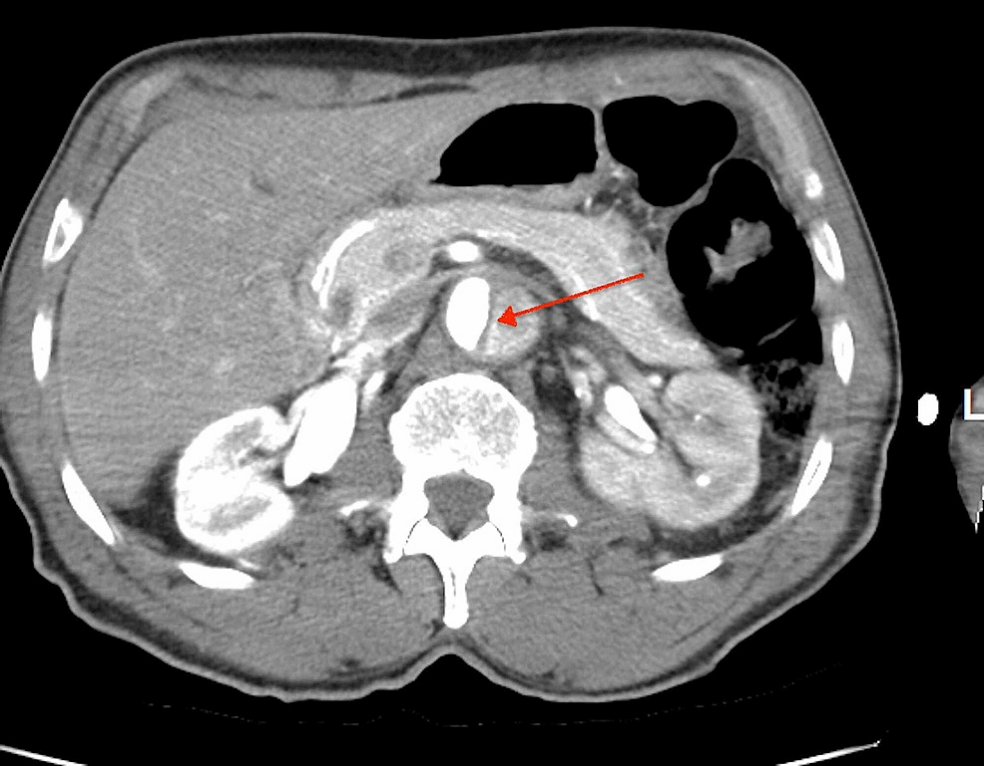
CT abdomen showing type B aortic dissection with true and false lumens separated by an intimal flap at the level of kidneys (renal arteries).
